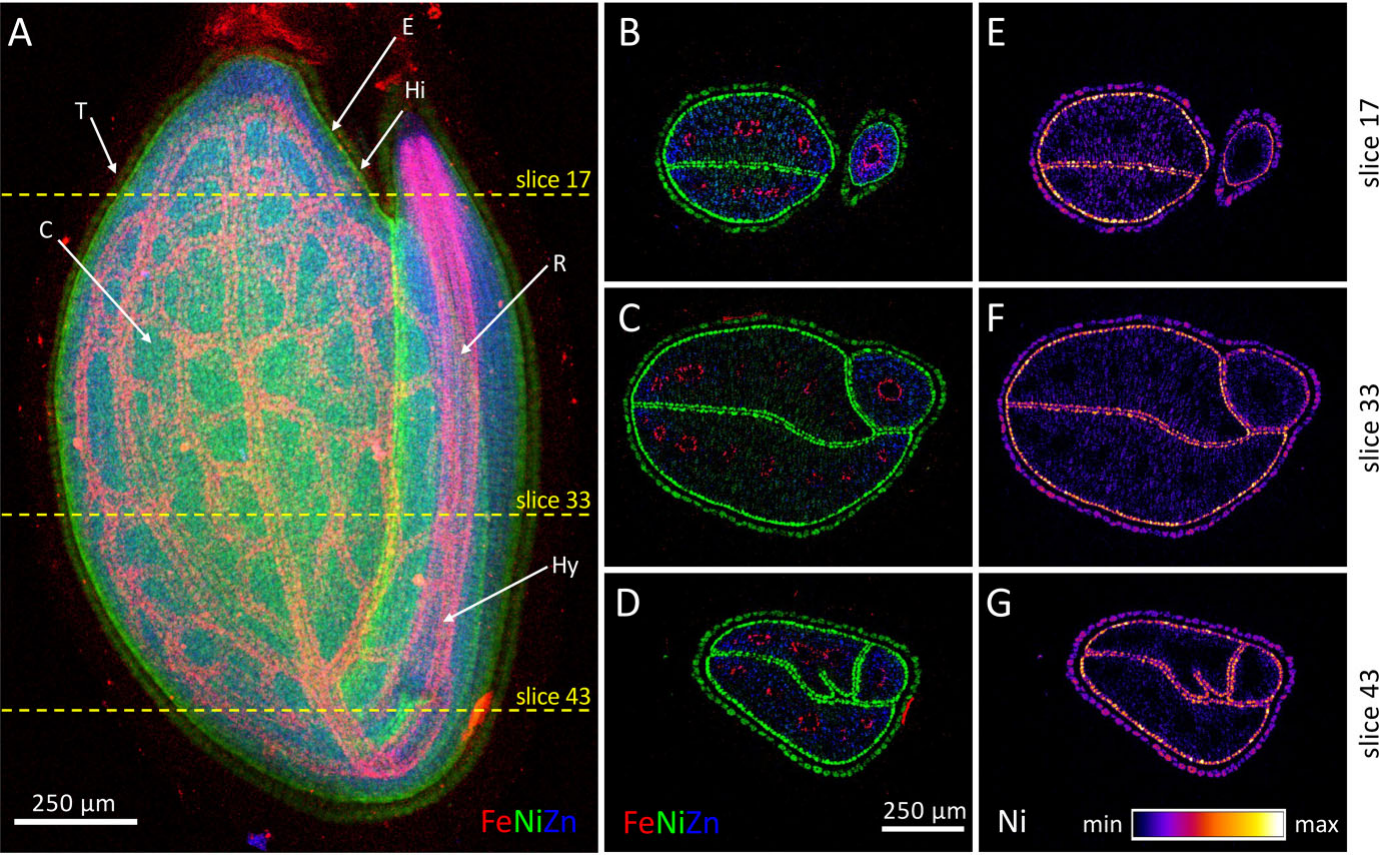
Synchrotron 2D projection map (A) and XFM–CT reconstructions (B–G) of a *Noccaea caerulescens* seed. A total of 51 tomographic slices were collected through the height of the seed; the selected XFM–CT reconstructions for slices 17, 33, and 43 show the distribution of Fe, Ni and Zn (B–D) and Ni intensity (E–G) T, testa, Hi, hilum, E, endosperm, R, radicle, Hy, hypocotyl, C, cotyledon. Reanalysed from source data presented in van der Ent *et al.*^72^
